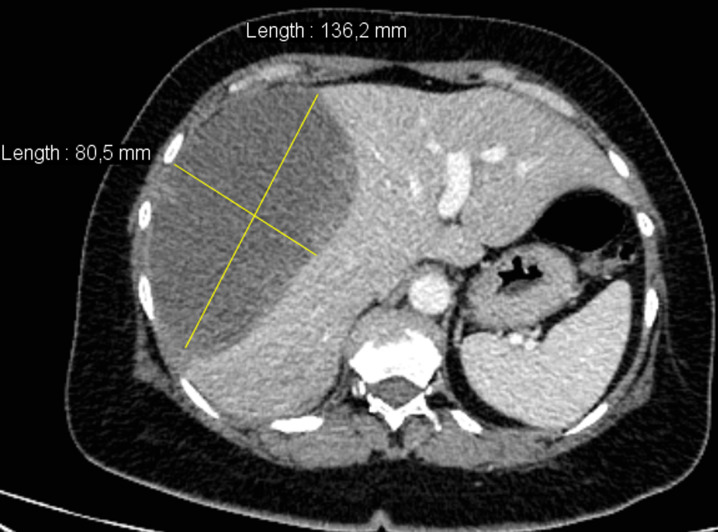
CT-scan showing sub-capsular hepatic hematoma, developed at the expense of segments VI, VII, and VIII of 136 x 80 mm in diameter
